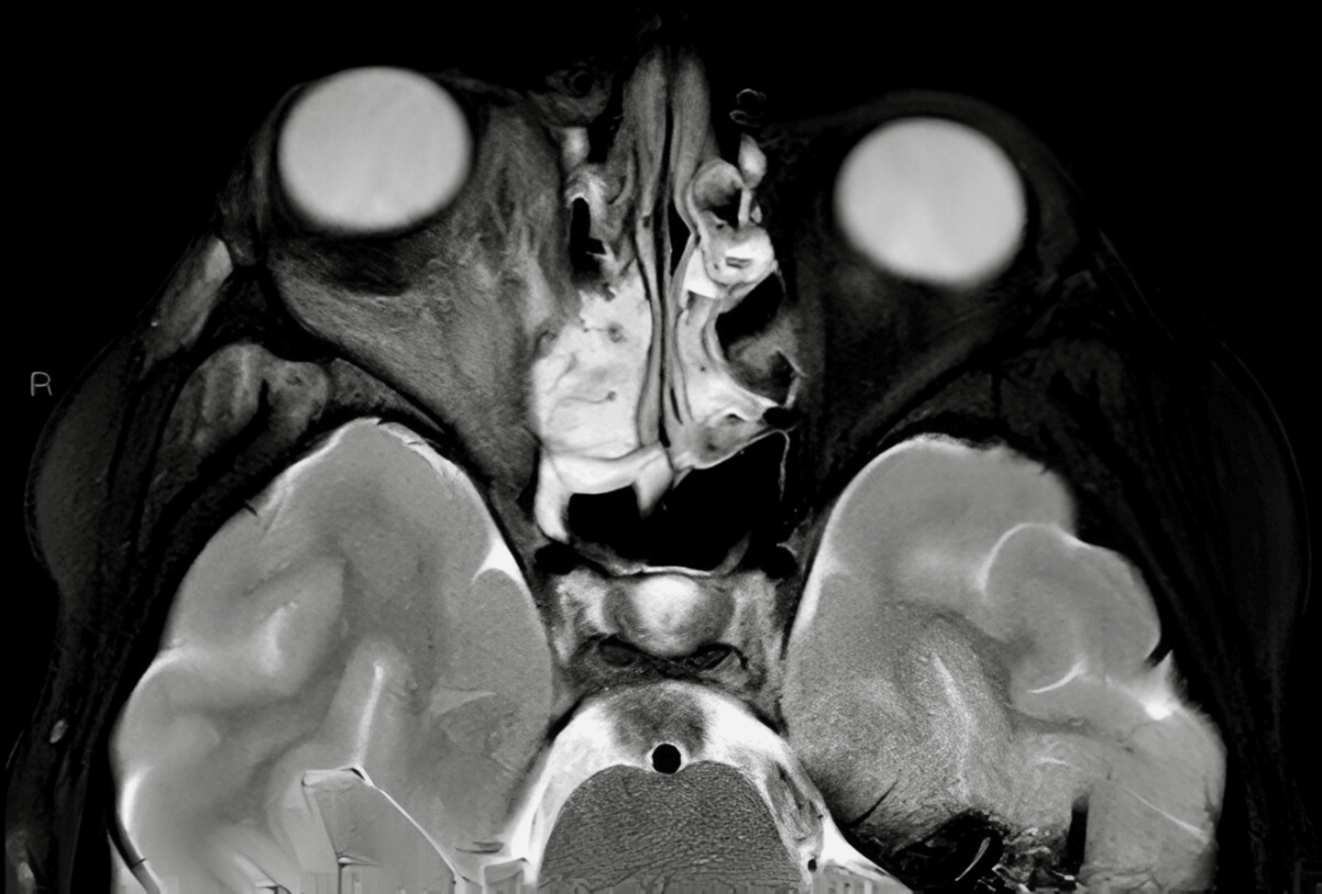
T2-weighted MRI of the brain showing right sided proptosis and widespread inflammation
